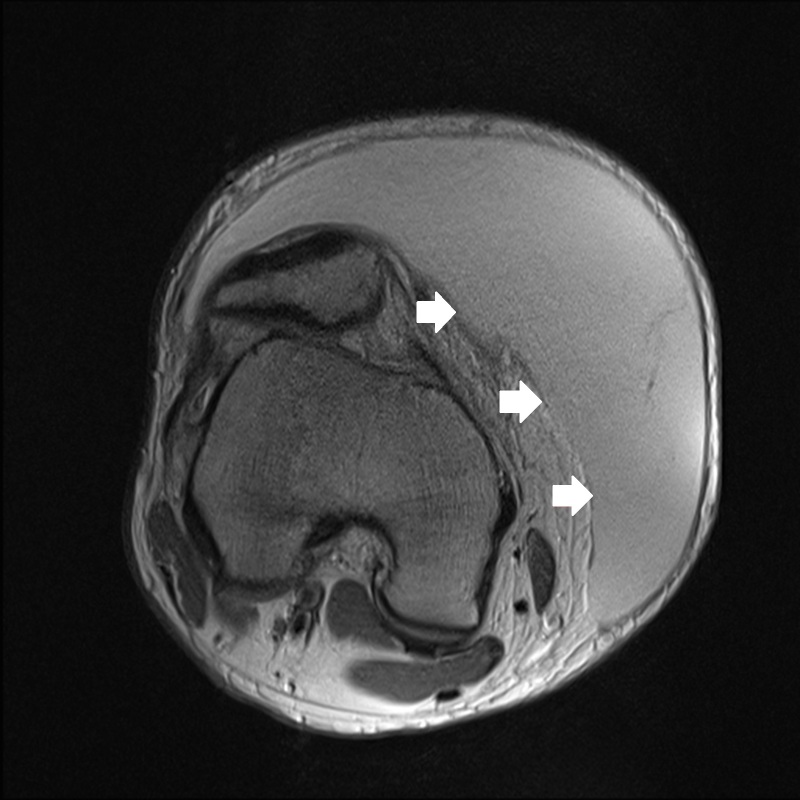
Axial proton density high resolution magnetic resonance imaging shows T2 prolongation in the Morel-Lavallee lesion of the anteromedial right thigh soft tissues.
